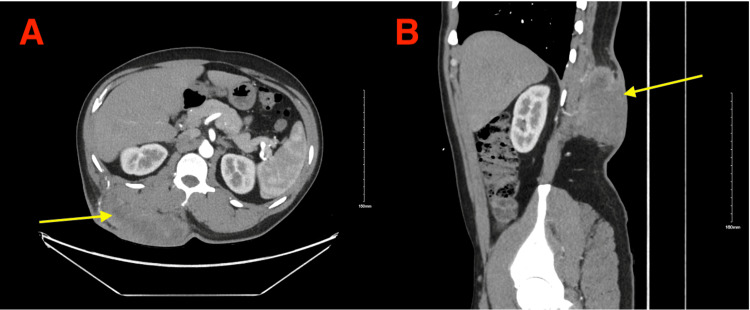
CT Scans Taken Following Radiation Therapy Post-radiation therapy axial (A) and sagittal (B) CT sections showing the extent of the mass (yellow arrows) in the right lumbar region of the back. CT: computed tomography
